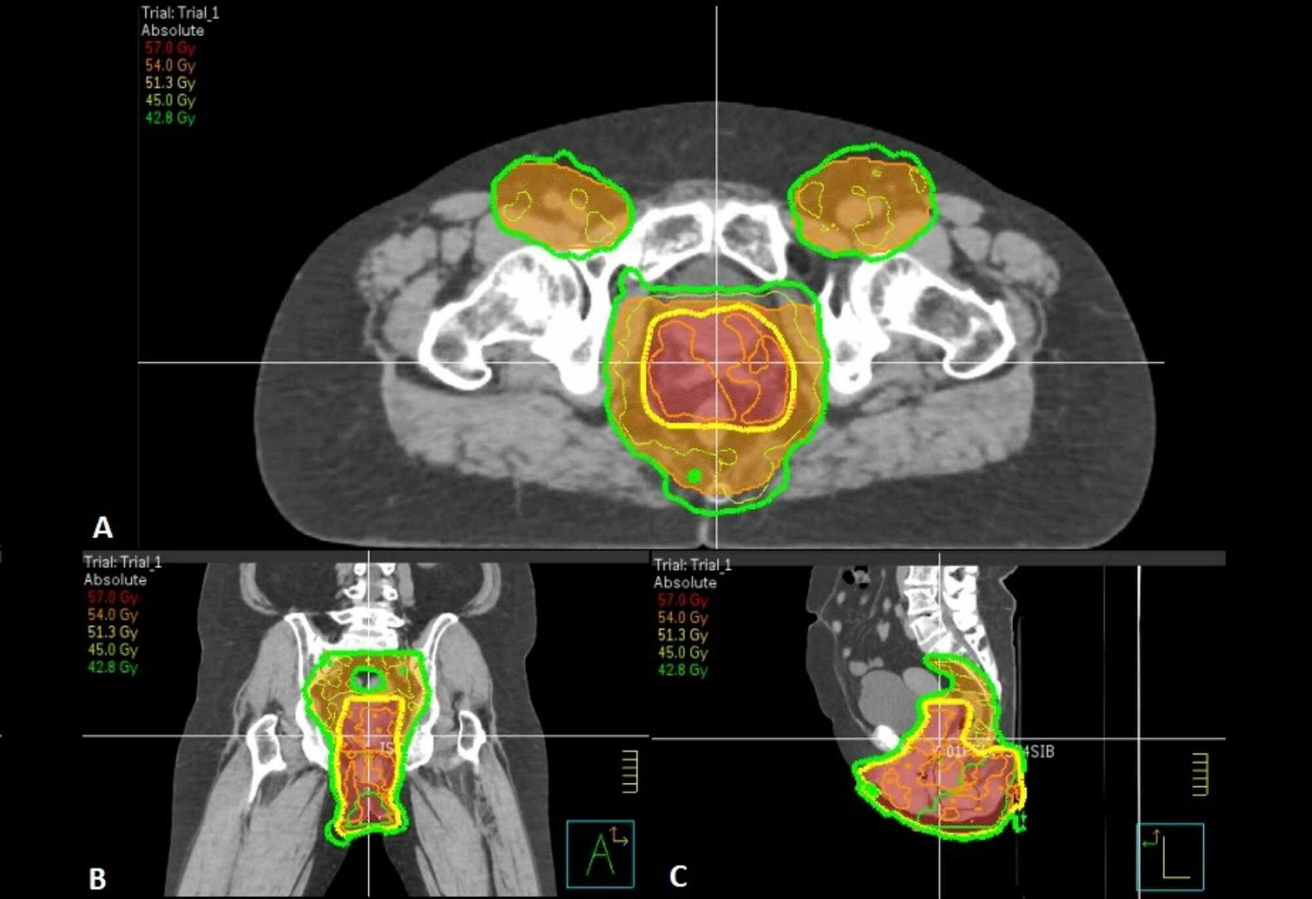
Dosimetric plan showing the various doses within the pelvis in Gy, with the corresponding colour legend located on the top-left hand corner CT planning scan in A) axial plane, B) coronal plane, and C) sagittal plane CT: computed tomography
